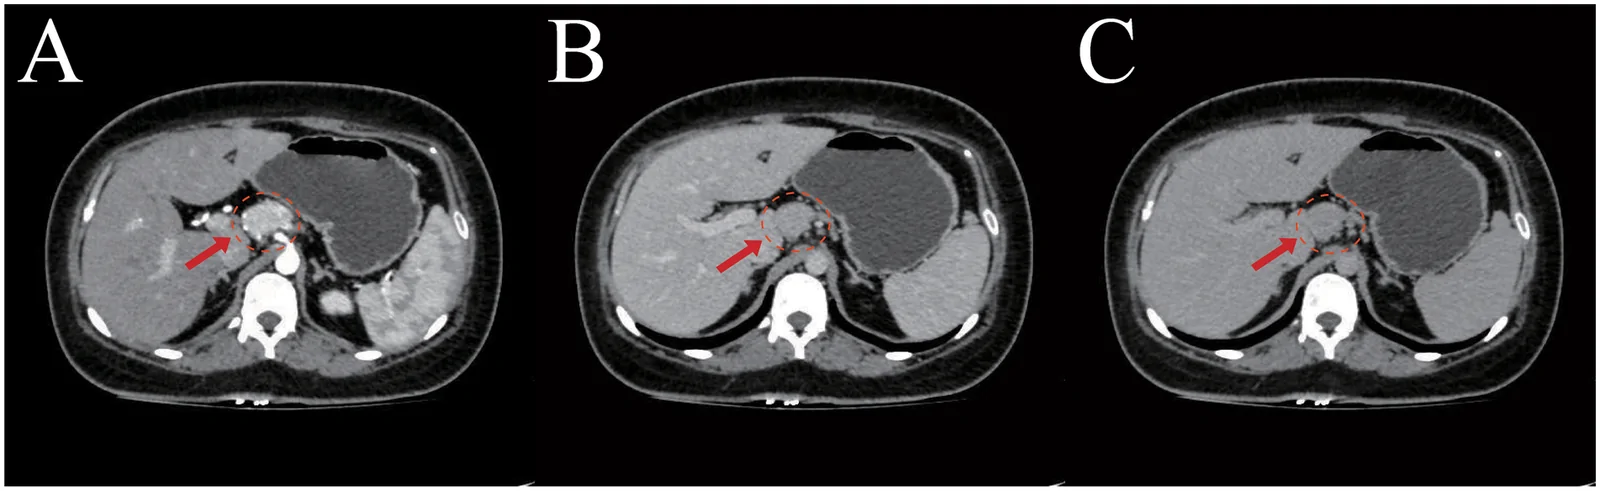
Contrast-enhanced abdominal CT findings. (A) Arterial phase showing a round, well-defined hypervascular mass at the superior border of the pancreatic body (mean attenuation, 146.7 HU). (B) Portal venous phase showing persistent but lower enhancement (mean attenuation, 96.3 HU). (C) Delayed phase showing further decreased attenuation (mean attenuation, 75.3 HU). Red arrows and dashed circles indicate the lesion.
